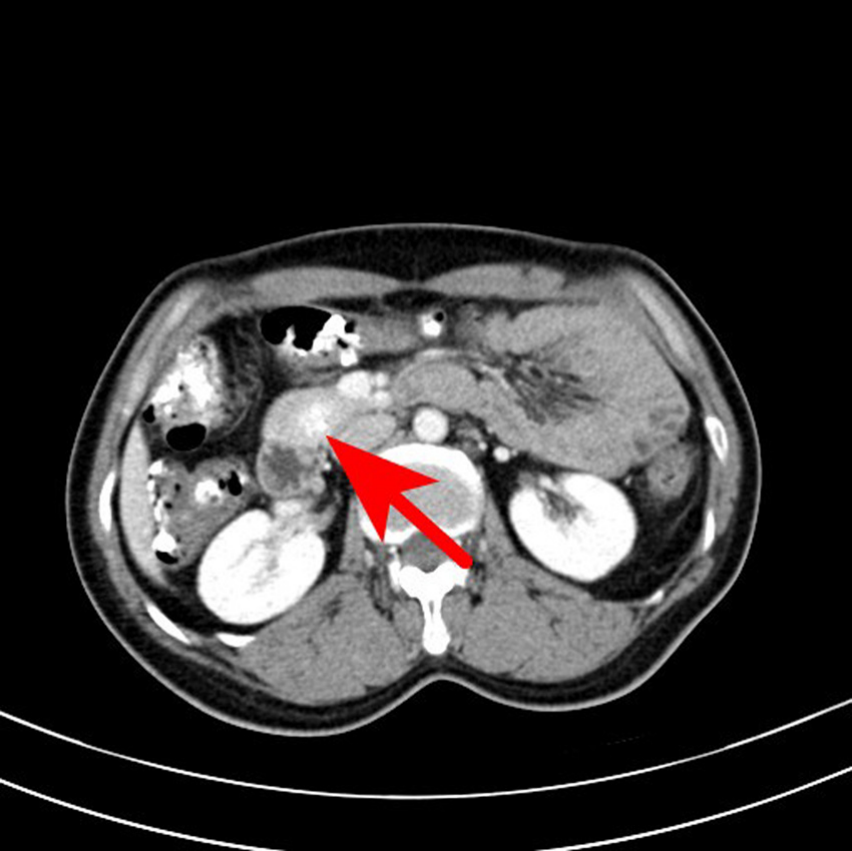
Transverse axial scan of the portal venous phase. The lesion was still high-density with CT value of 174 HU.
